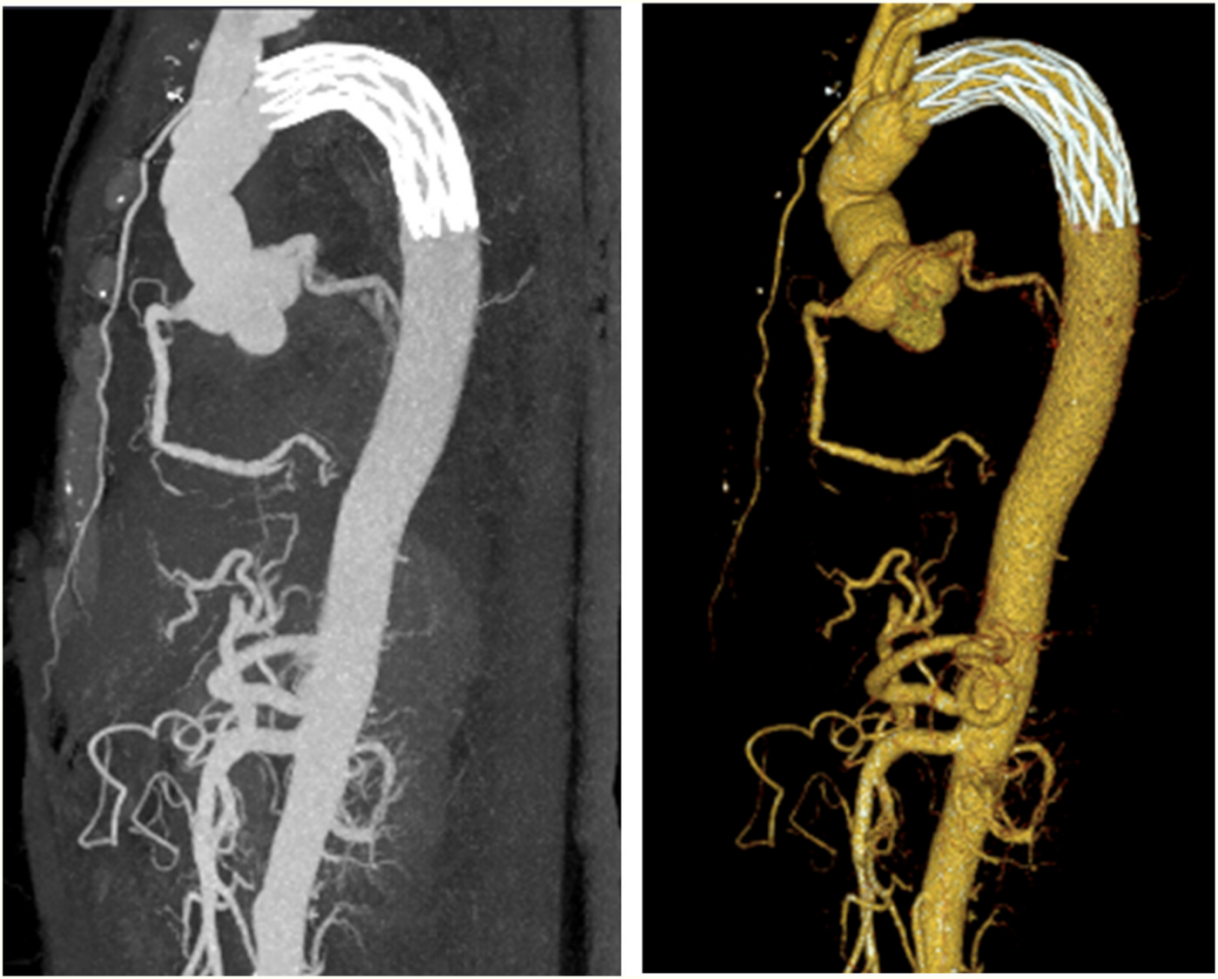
Computed tomography conducted after aortic arch artificial vessel replacement.
